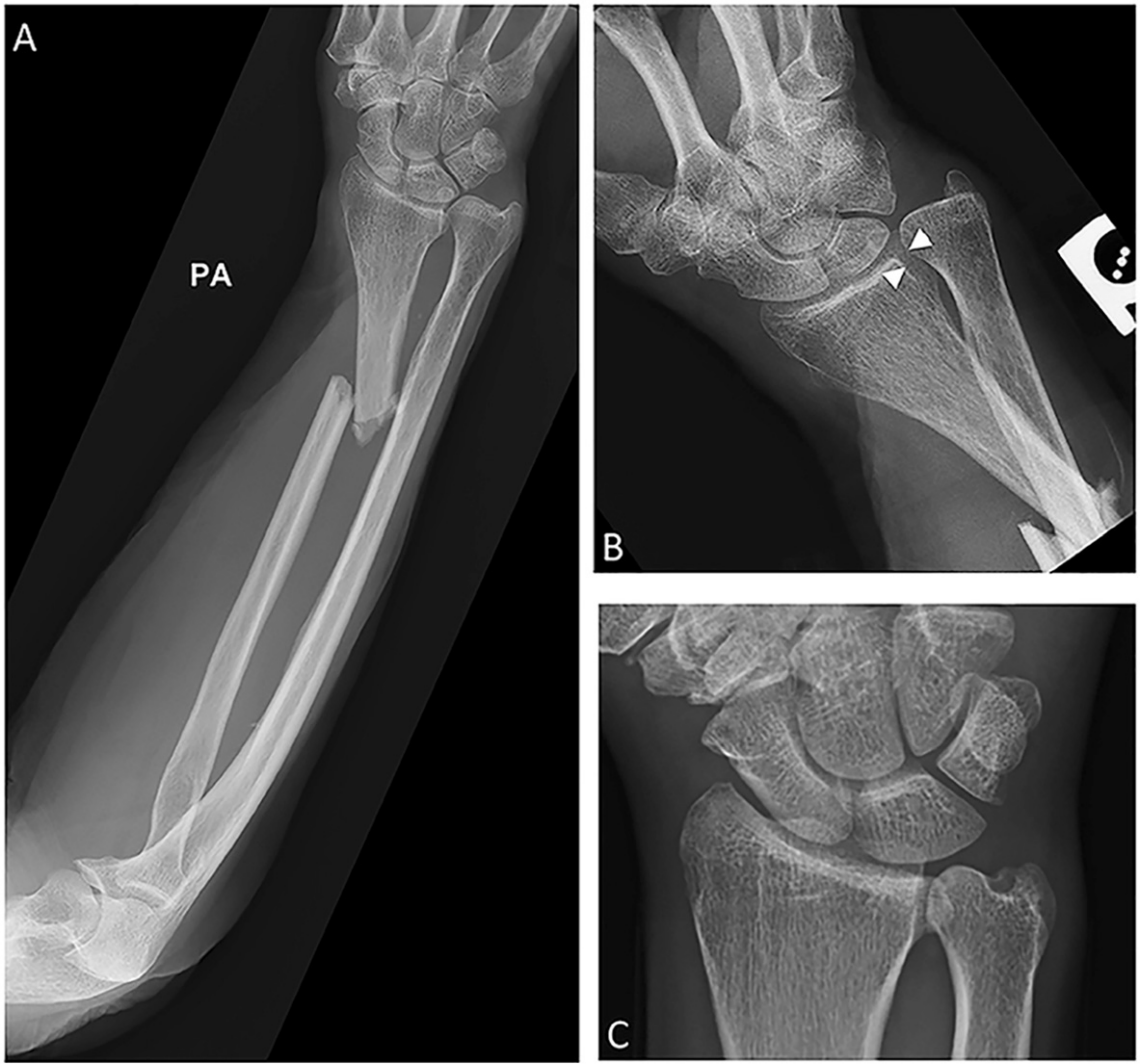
Galeazzi fracture. a PA forearm radiograph with displaced fracture of the distal one third of the radial shaft. b Wrist radiograph in the same patient demonstrates subluxation of the distal radioulnar joint (DRUJ), with mild DRUJ widening, measuring 5 mm (*arrowheads*), and mild radial foreshortening. c Comparison normal wrist radiograph. Notice the small caliber of a normal, tight DRUJ
